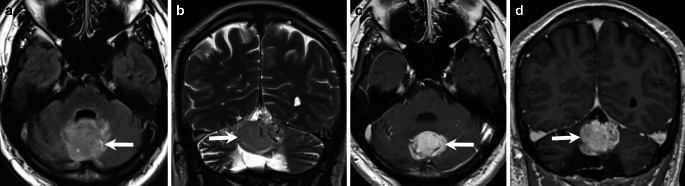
Bi-annual MRI follow-up from 2024 in the asymptomatic patient. On axial FLAIR images (a) and coronal T2-weighted images (b), the resection defect was filled with a lesion that appeared hyperintense compared to the surrounding cerebellar parenchyma (a,b, *arrow*). On axial (c), and coronal (d) T1-weighted images post-Gd administration, the lesion exhibited homogeneous contrast enhancement (c,d, *arrow*)
